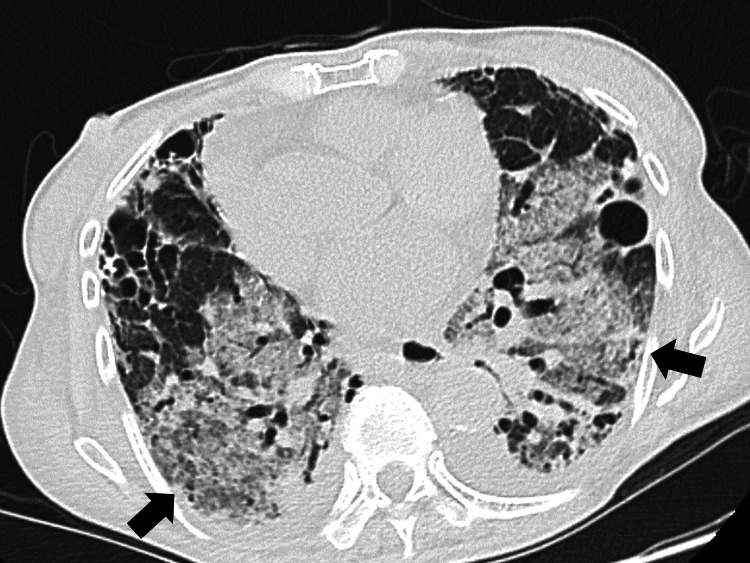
Computed tomography image showing interstitial infiltration in bilateral lungs.
